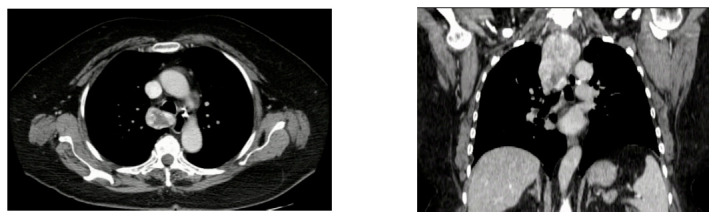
Axial and coronal CT scan of a patient with an 8 cm intrathoracic goiter not amenable to US-guided FNA.
